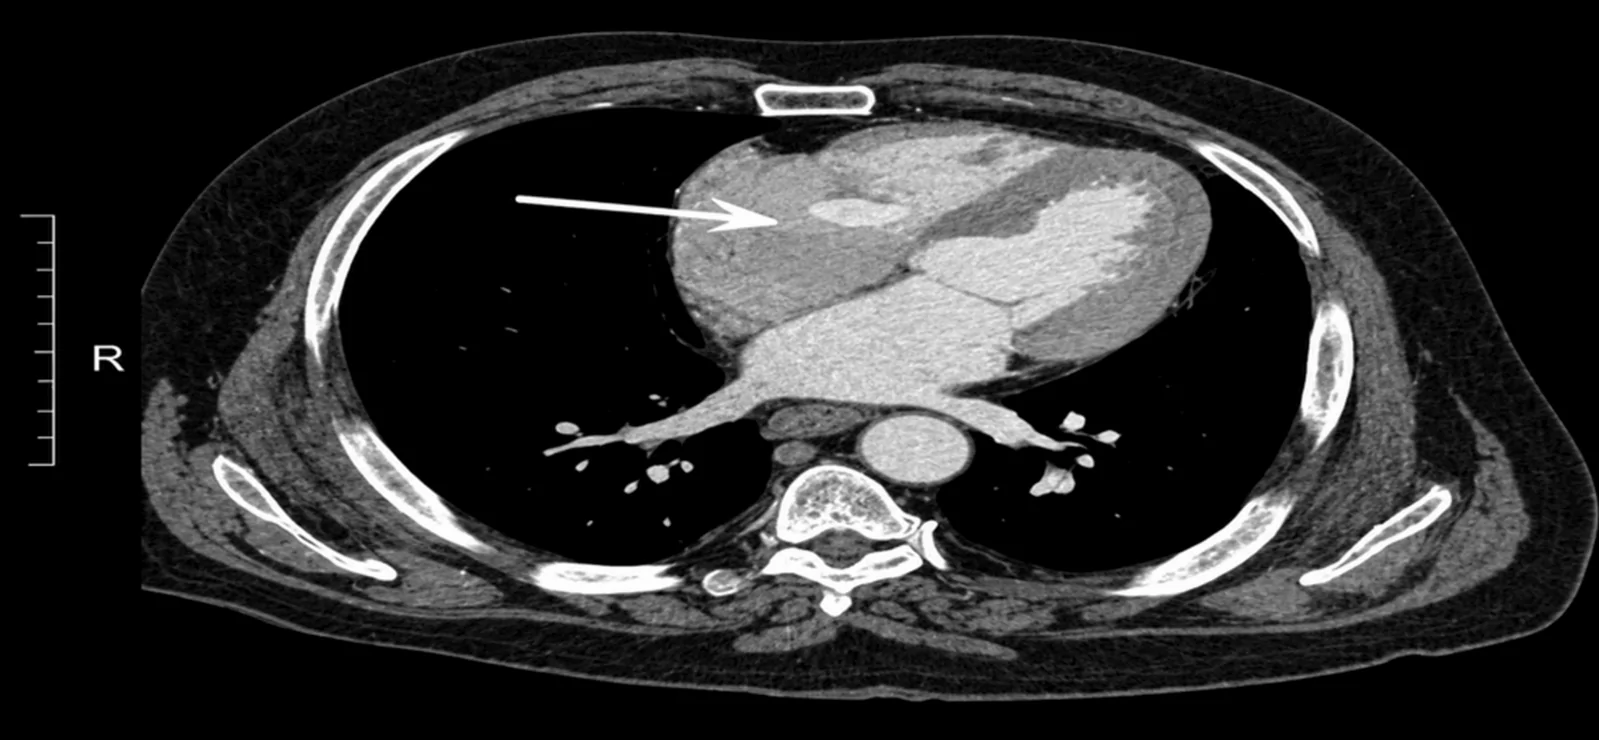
Chest computed tomography angiography showing a migrated venous stent within the right-sided cardiac chambers. The arrow indicates the migrated stent projecting across the right atrium and right ventricle.
